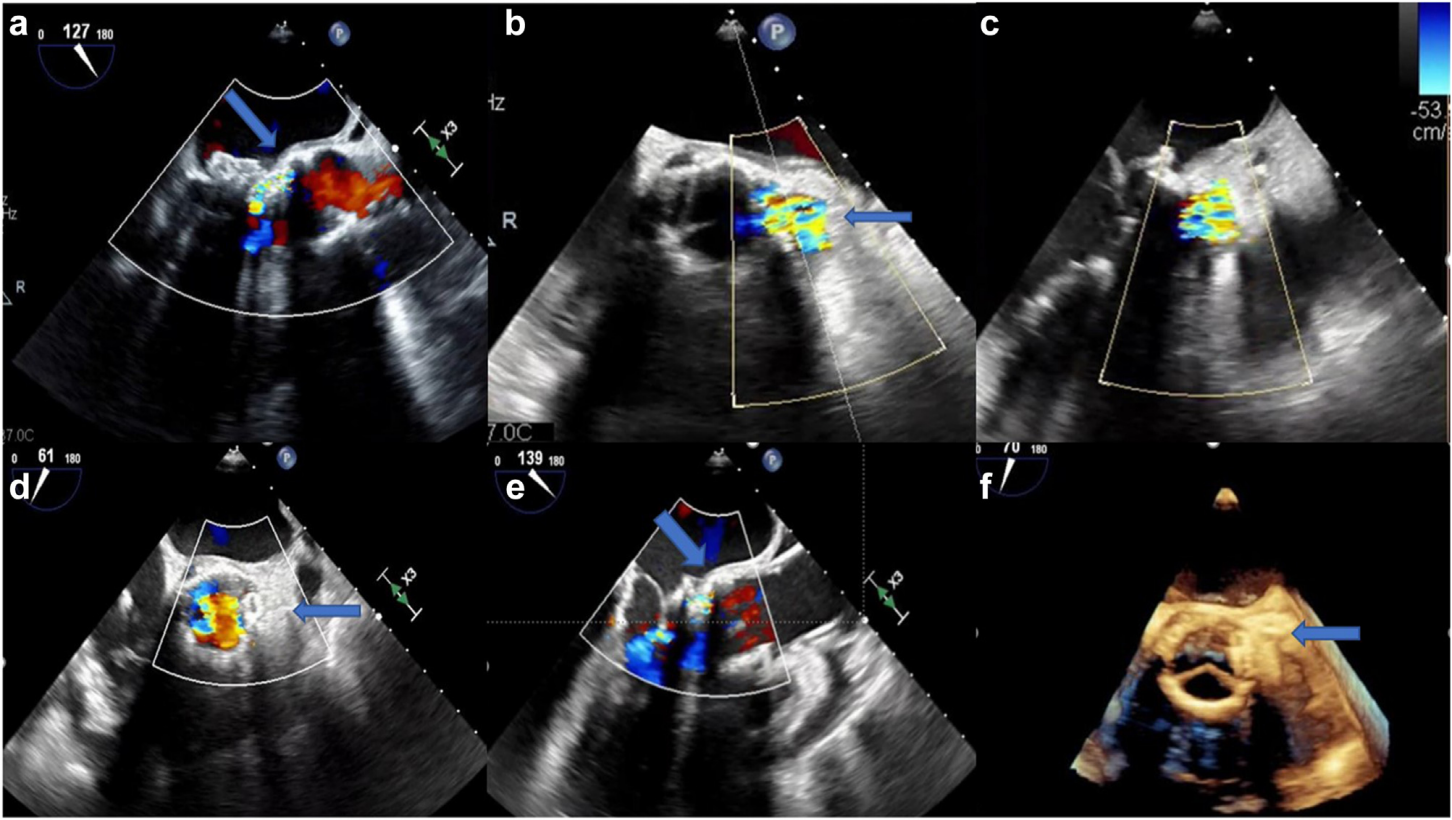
TEE 6 weeks after index procedure (SITRAL-TAVR) demonstrating pseudoaneurysm from aorta to LV adjacent to aortic Sapien transcatheter valve (arrow) (a-c). Postintervention TEE demonstrating Amplatzer Plug (arrow) and coiling of pseudoaneurysm with reduced flow around Sapien transcatheter valve (d-f). Abbreviations: TAVR, transcatheter aortic valve replacement; TEE, transesophageal echocardiogram.
